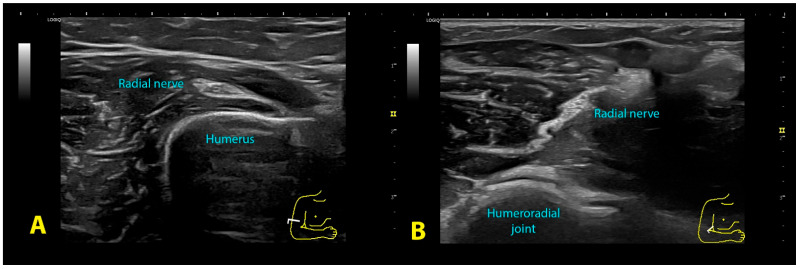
Ultrasound image of the radial nerve at the spiral groove (A) and the antecubital fossa (B).
